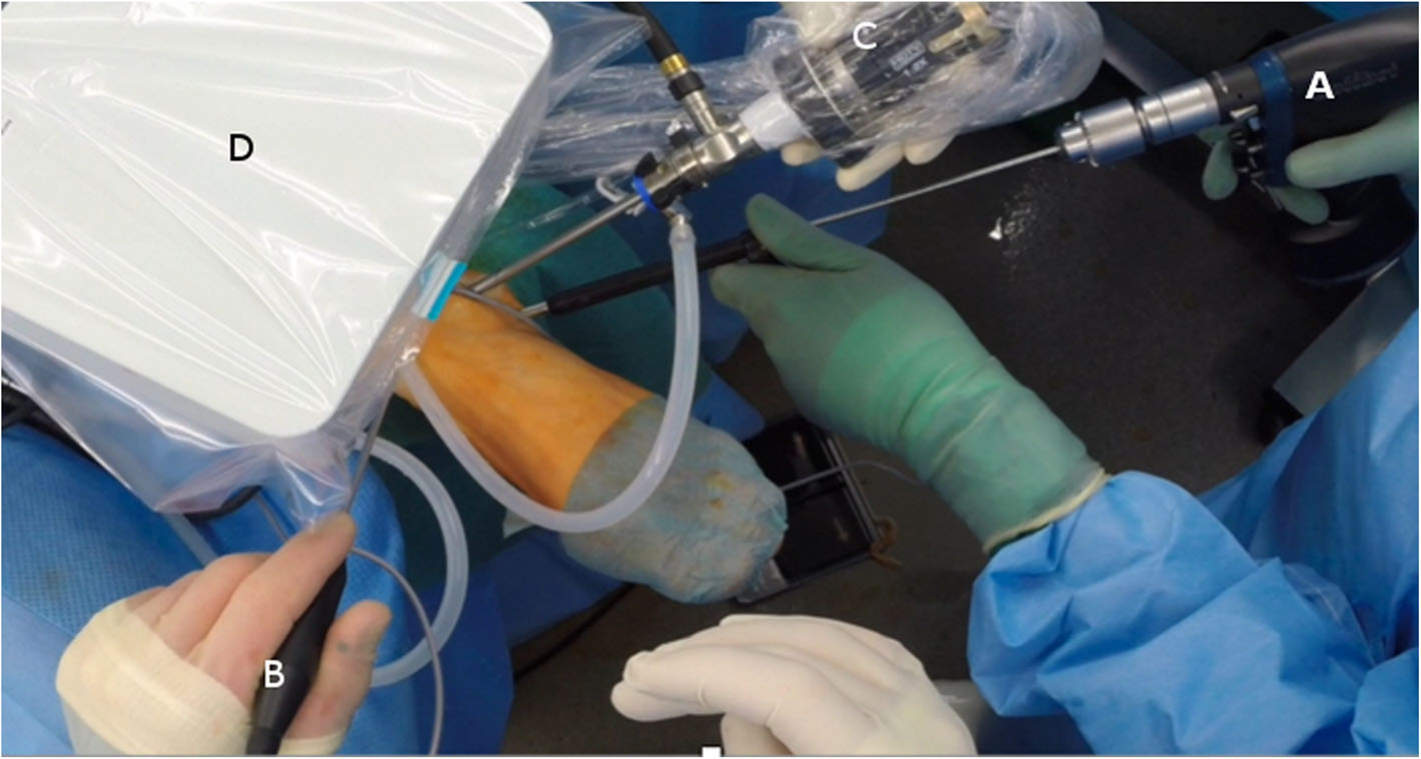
Intraoperative setting; a Drill, b Probe hook, c Arthroscope, d x-ray
